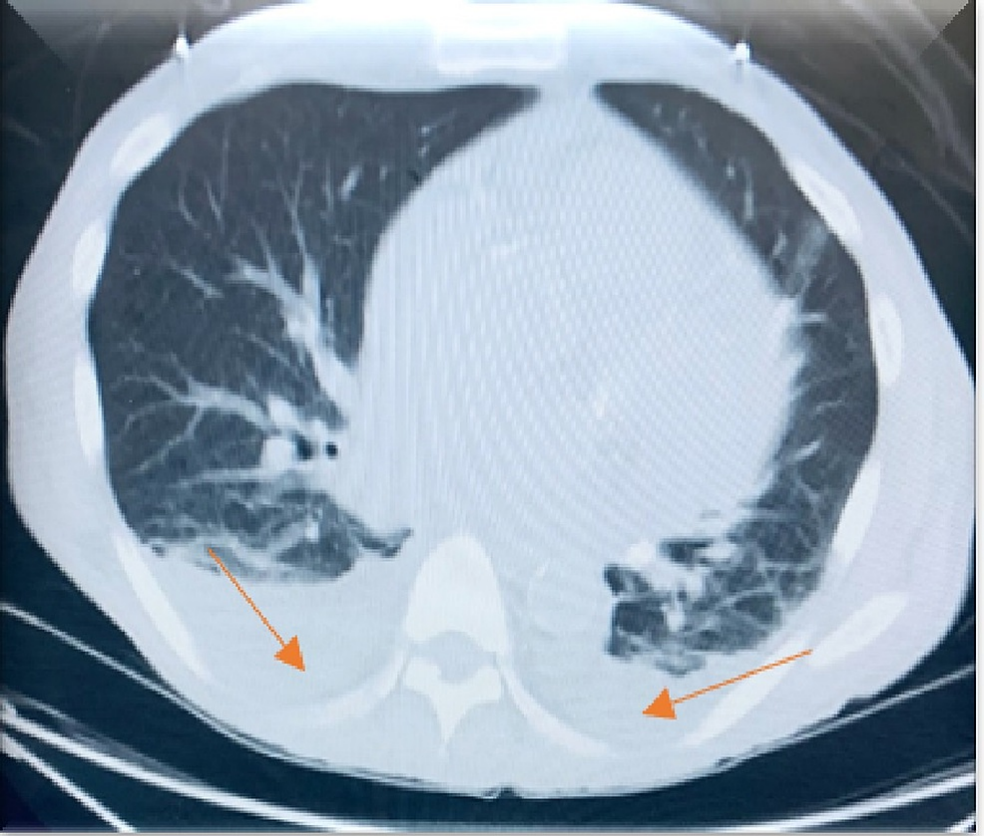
CT chest lung window showing bilateral pleural effusion.
